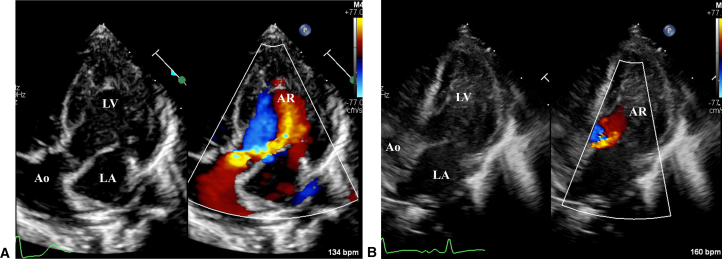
A, Preoperative transthoracic echocardiography showing severe aortic regurgitation. B, Postneocuspidization transthoracic echocardiography showing mild aortic regurgitation. *LV*, Left ventricle; *LA*, left atrium; *Ao*, aorta; *AR*, aortic regurgitation.
